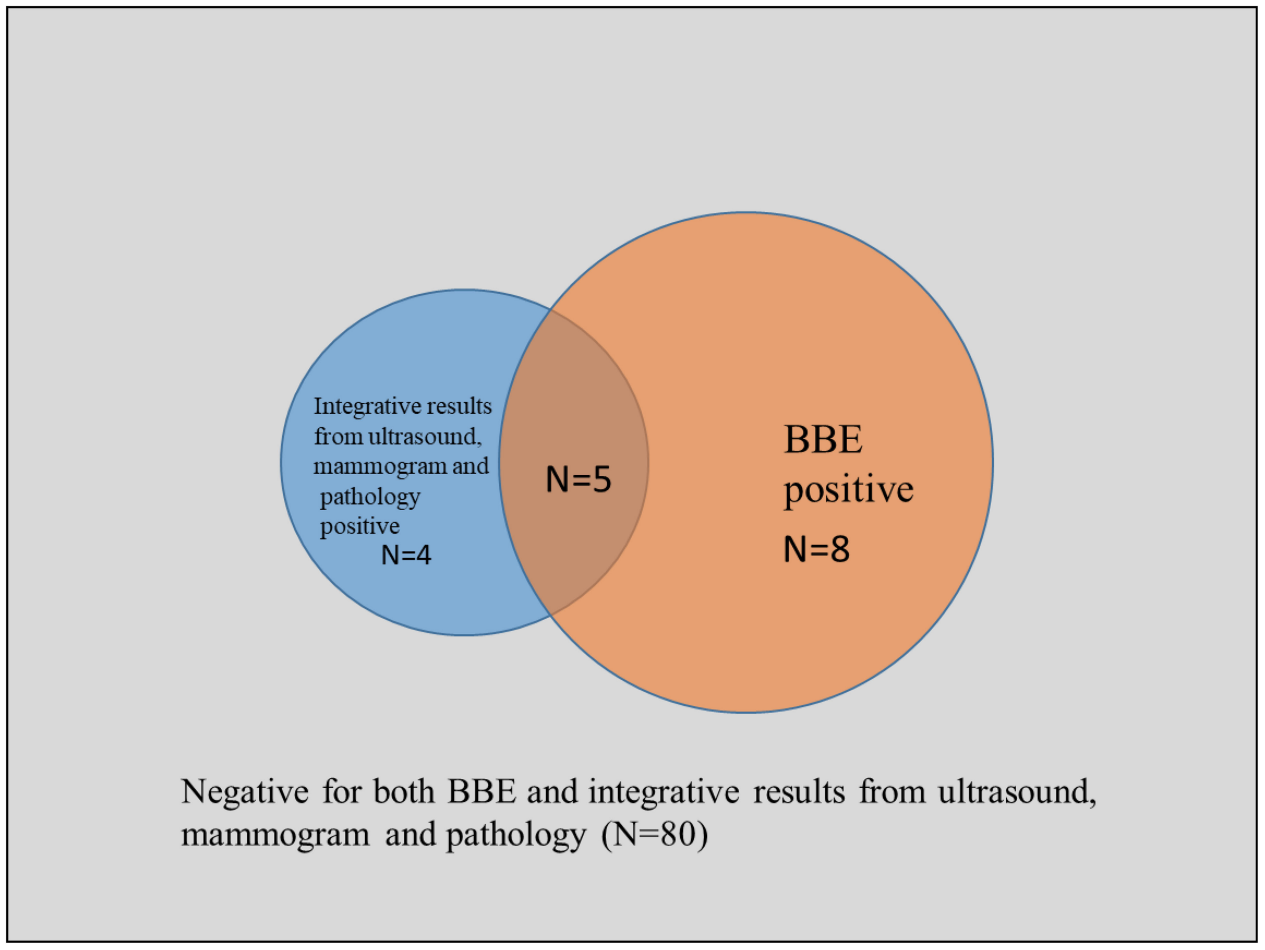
Venn plot of the detection results from BBE and integrative results from ultrasound, mammogram and pathology.
